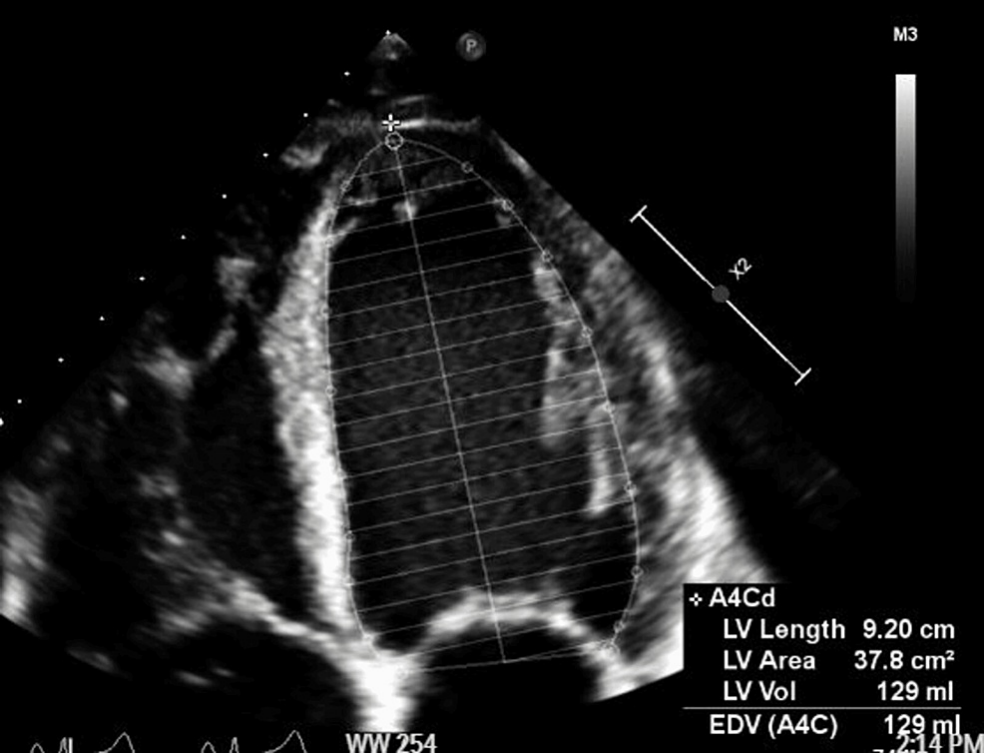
Apical four-chamber view in diastole revealing left ventricular measurements
